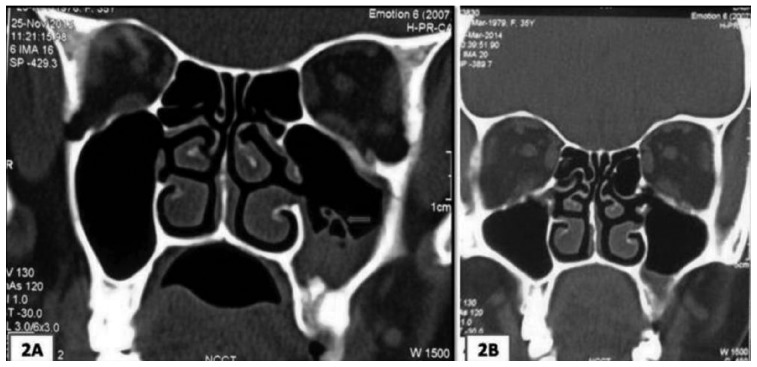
A) Contrast enhanced CT showing mucosal thickening in left maxillary sinus and radiopaque mass in the periapical region of 25. Irregular bony margins and discontinuity in the floor of the left maxillary sinus can also be noted. B) Post-operative CT scan showing marked reduction in mucosal thickening in the left maxillary sinus at 3-month follow up.
